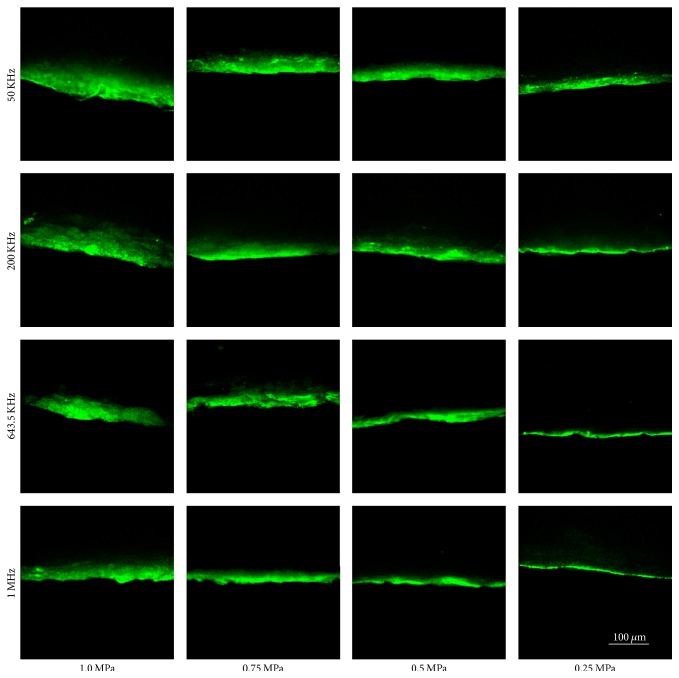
The confocal images of penetration depth at different frequencies and intensity under the influence of ultrasound alone for 10 mins.
